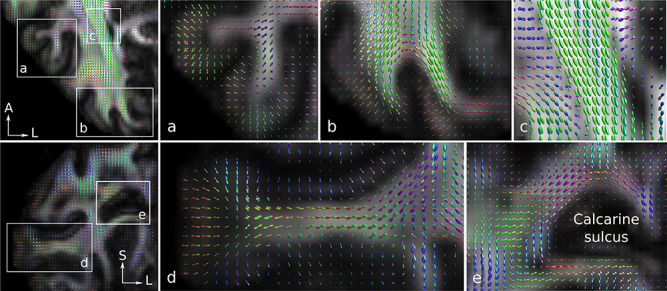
Robust fODF estimates were enabled using submillimeter resolution DWI and MSMT-CSD. The flexible surface coil fODF estimates are shown for a representative participant and are superimposed on the DWI-derived fractional anisotropy map. A, S, and L: anterior, superior, and left, respectively. In a selected axial slice, (*a*) intracortical radial fibers running perpendicular to the cortical GM boundary in the gyri and along the walls of the sulci were detected (see Supplementary Video 1). (*b*) U-fibers running parallel to the cortical GM boundary are shown in a sulcus in the early visual cortex. U-fibers form crossing regions with long-range fibers (Reveley et al. 2015) and obscure their penetration into the cortical GM using submillimeter spatial resolution DWI techniques (see Supplementary Video 2). (*c*) Optic radiation and the posterior tail of the splenium of the corpus callosum tracts were detected to run predominantly anterior–posterior with few or no secondary peaks as expected (see Supplementary Video 1). In a selected coronal slice, (*d*) intracortical radial fibers running perpendicular to the cortical GM boundary in the gyrus and along the wall of the sulcus were reconstructed (see Supplementary Video 3). (*e*) Fibers near the calcarine sulcus were detected to run predominantly parallel to the cortical GM boundary (see Supplementary Video 4).
